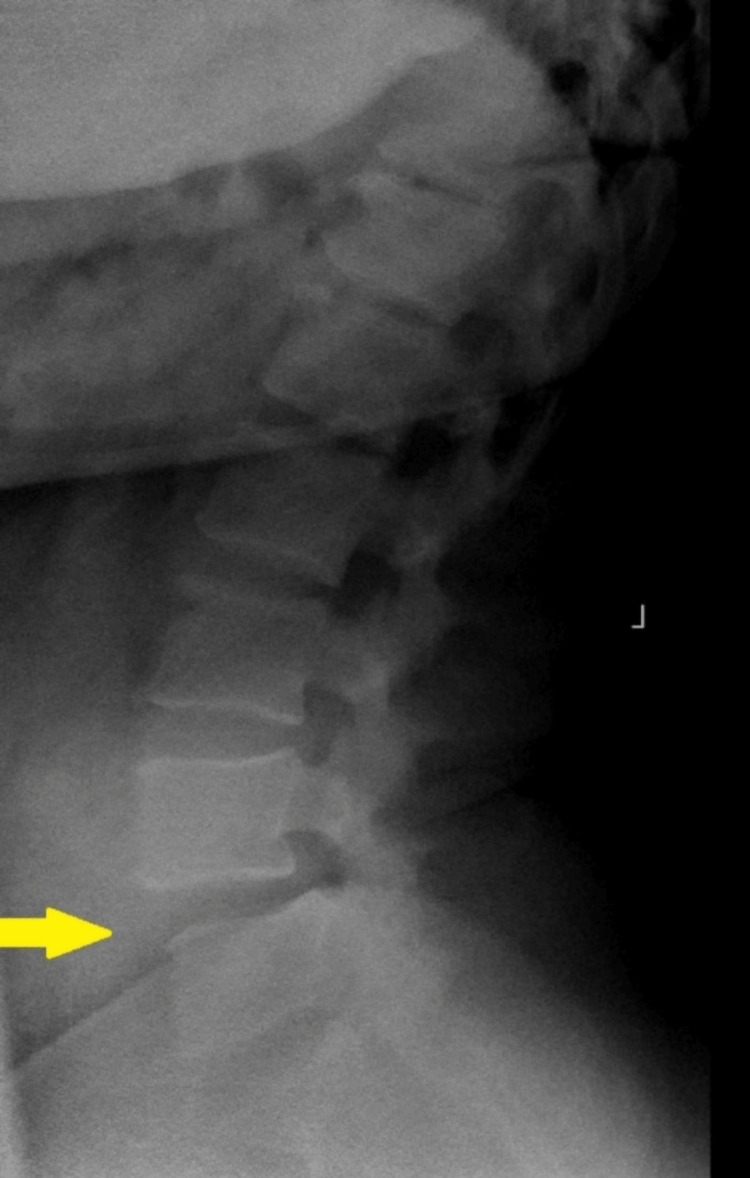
Weight-bearing lateral lumbar radiograph showing grade I anterolisthesis at L4-L5 intervertebral level (yellow arrow)
